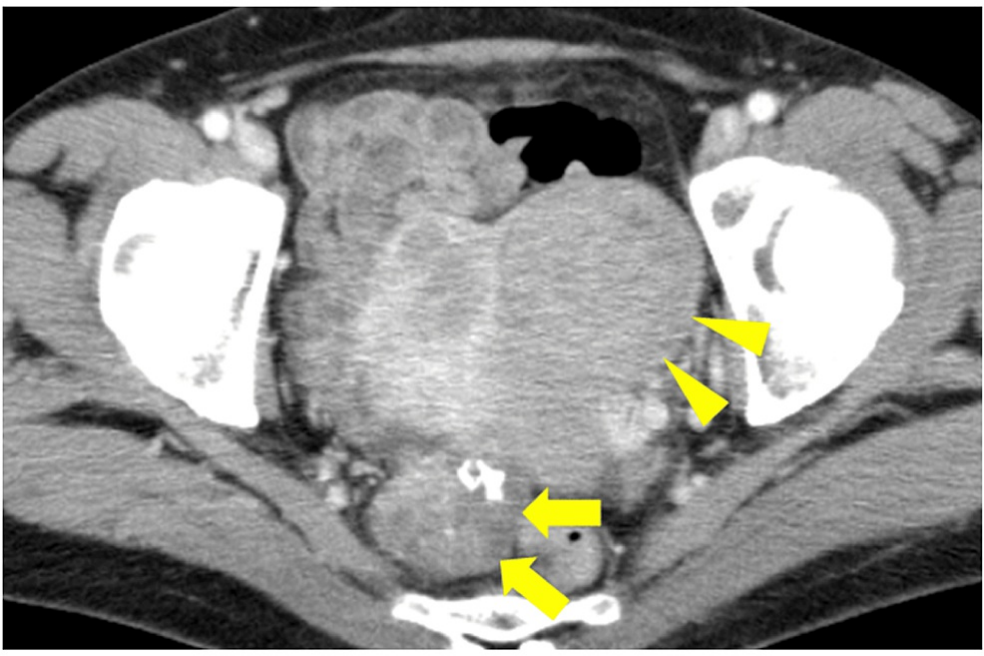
Abdominal pelvic contrast CT tomography performed two years prior to urological surgery. A mass with calcification (arrow) can be observed in the right dorsal pelvis. A uterine myoma observed on the ventral side (arrowhead) is thought to be the same as the uterine myoma at presentation (Figure 1).
